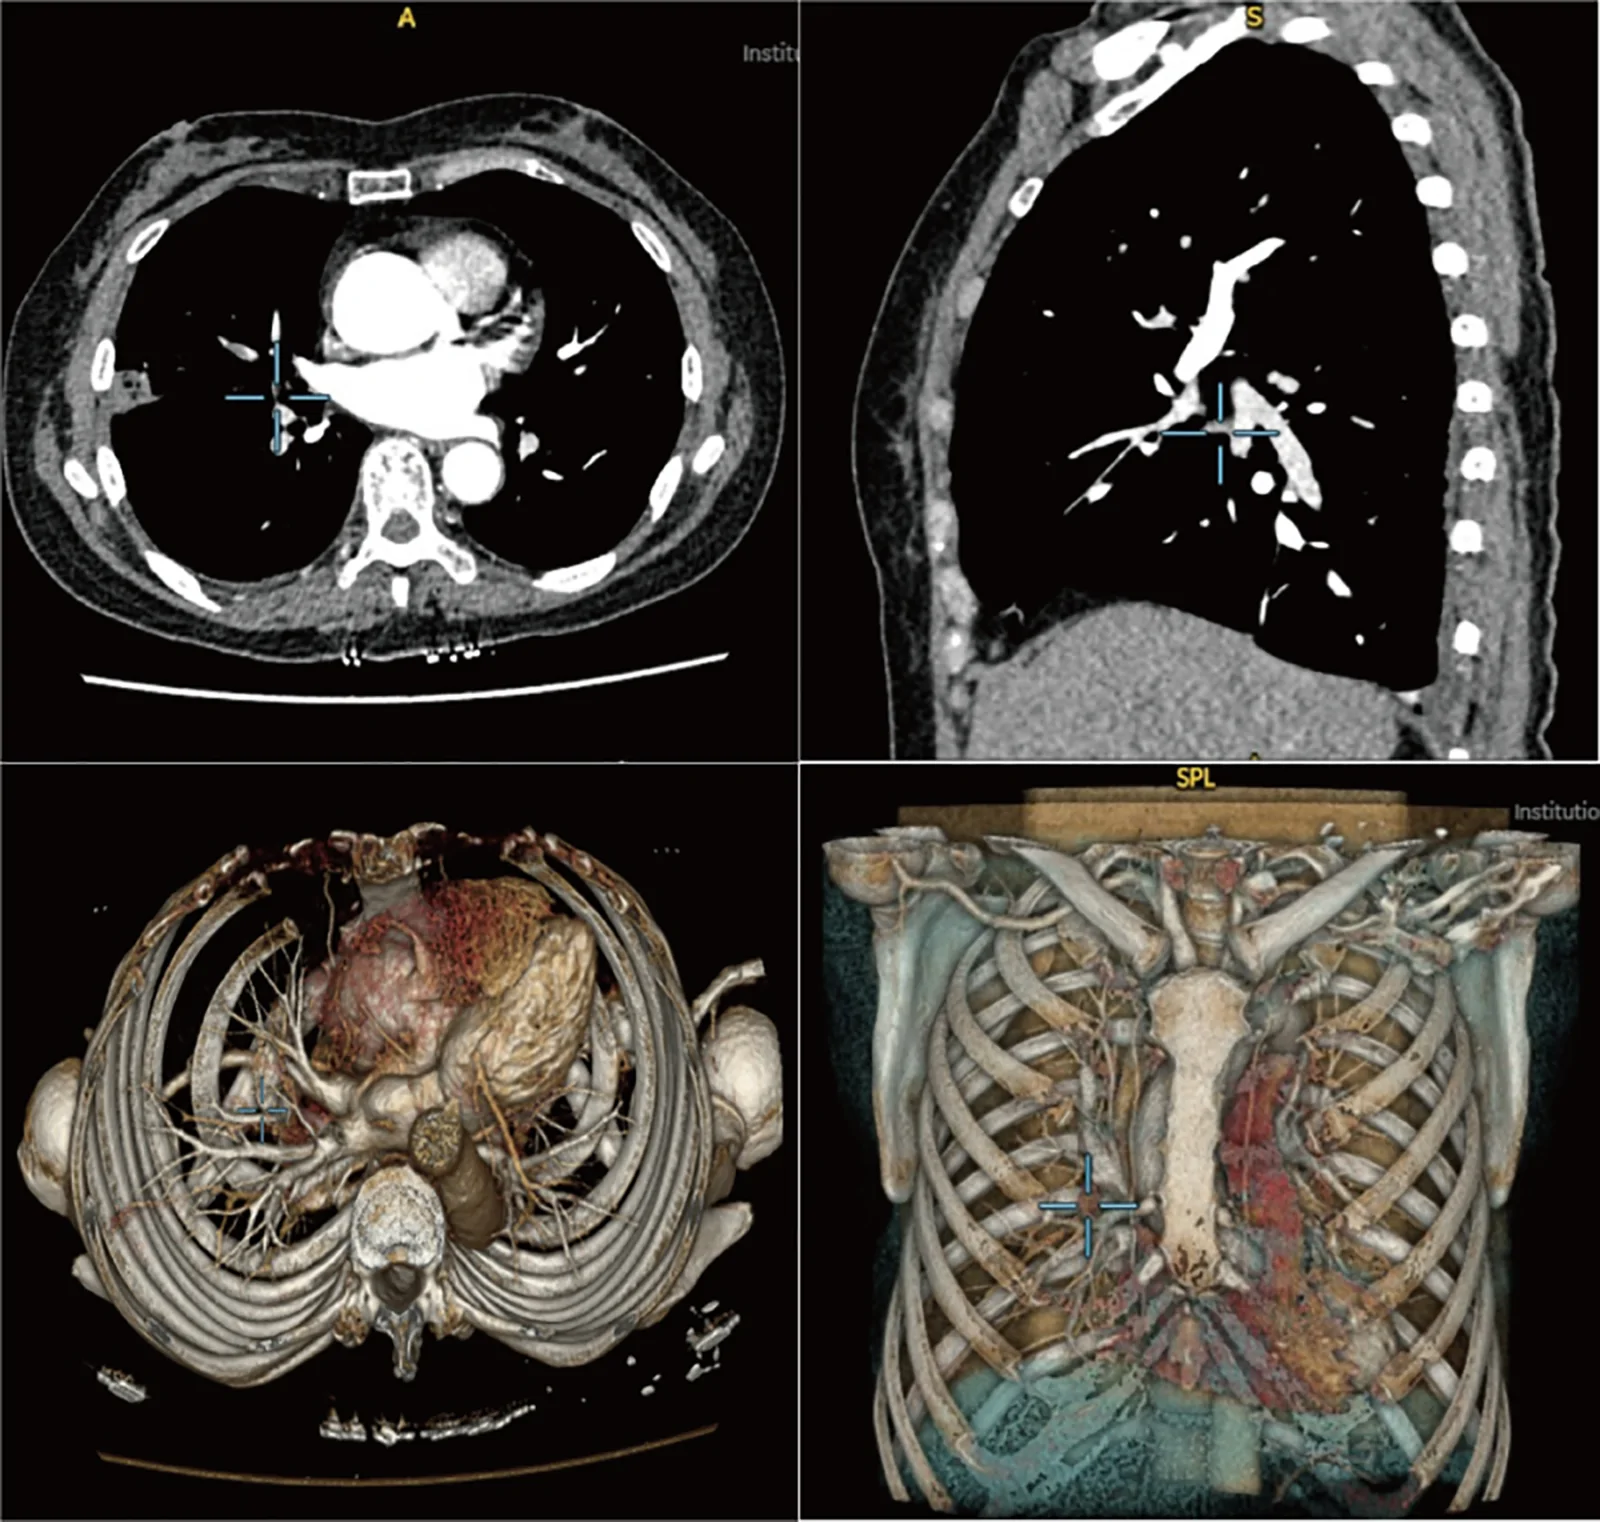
Pulmonary artery CTA results of a patient with mixed hemorrhoids following surgery, dated July 8, 2025. CTA = computed tomography angiography.
